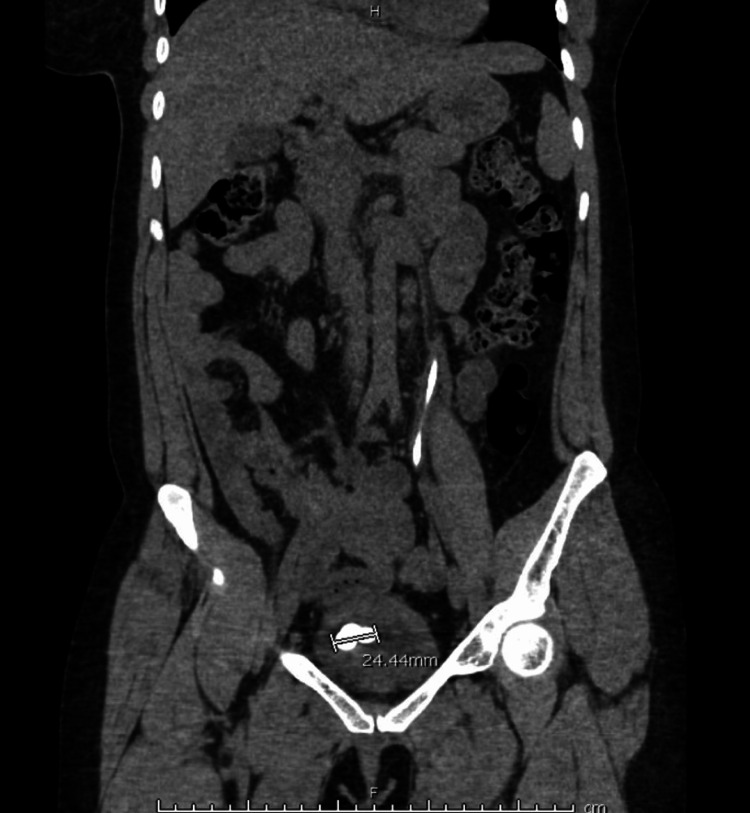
Pre-operative CT abdomen/pelvis further revealed the distally calcified ureteral stent in the urinary bladder
